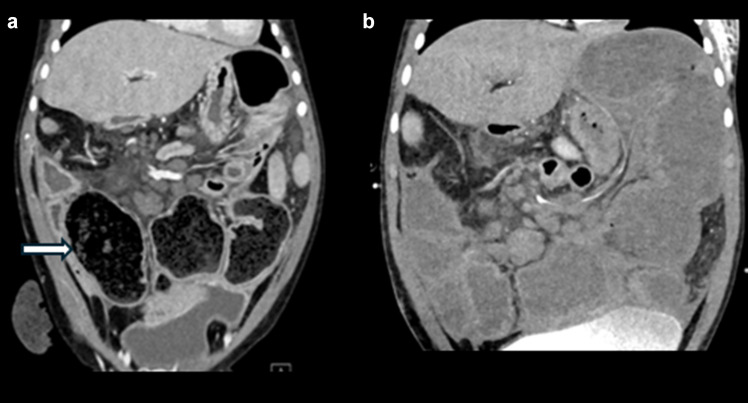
Coronal CT abdomen/pelvis with contrast image performed in a patient with MMIHS at age 5. (a) Dilated bowel loops proximal to the ostomy with fecalization of bowel contents (arrow), suggesting obstruction versus pseudo-obstruction. (b) Follow up coronal CT with contrast demonstrates persistent dilation of bowel loops proximal to the ileostomy, consistent with ongoing pseudo-obstruction. The patient frequently undergoes ileostomy irrigations
